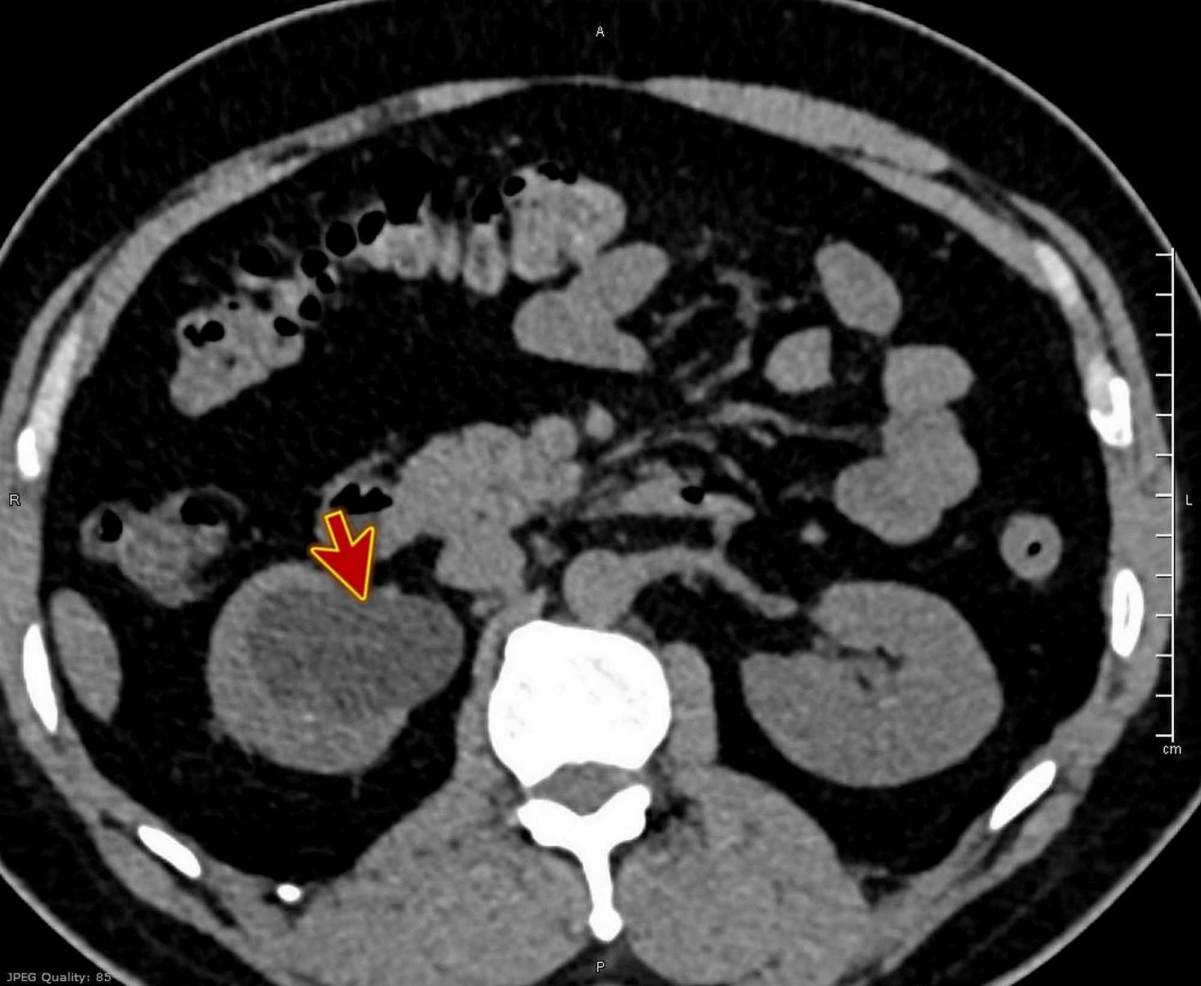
CT KUB showing right moderate to severe hydronephrosis (red arrow) and perinephric fat strandings. CT KUB, computed tomography of the kidney and the urinary bladder
